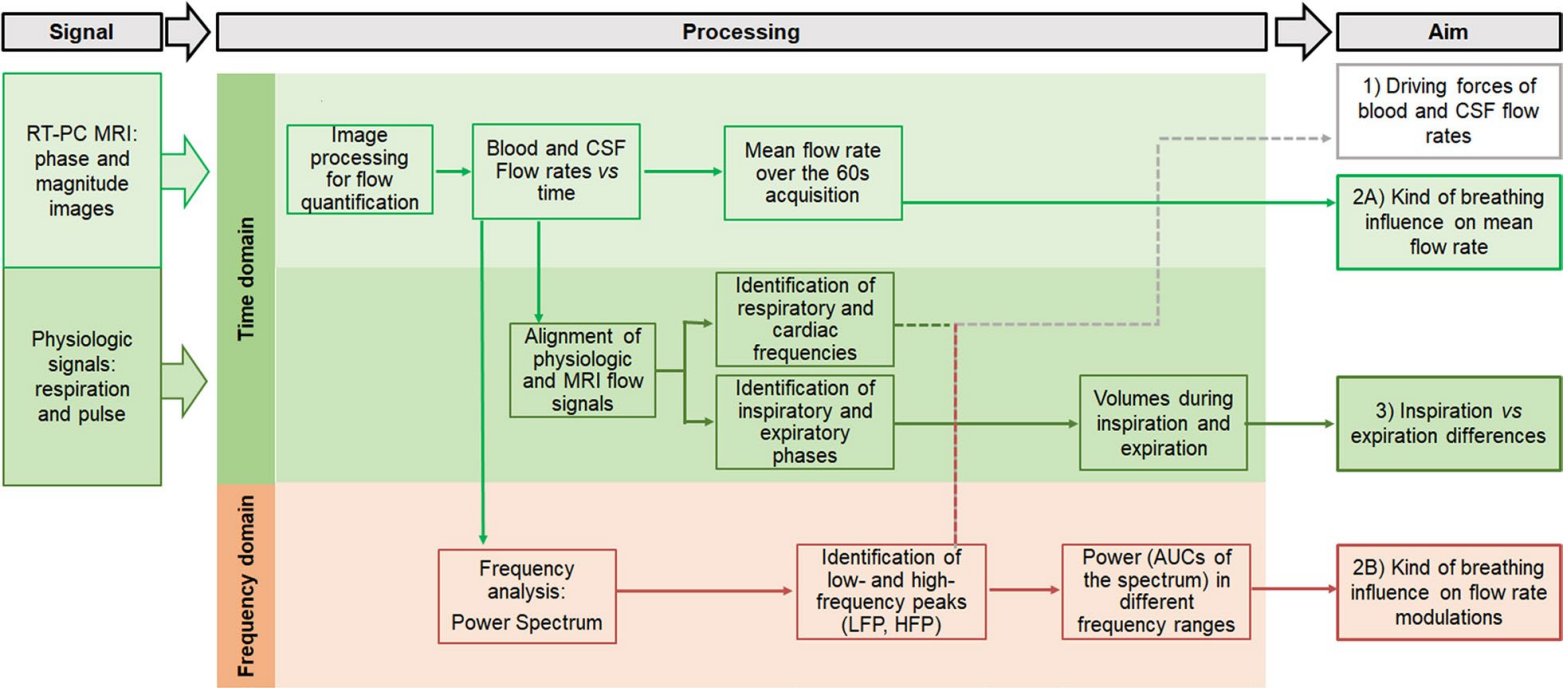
Scheme of the Real-Time Phase-Contrast (RT-PC) MRI data and physiological signals processing
